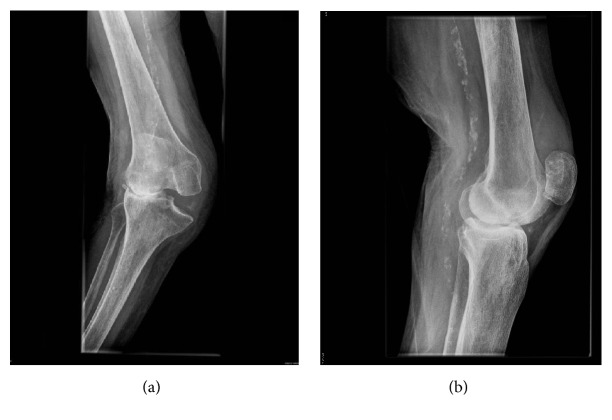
Preoperative standing X-rays of the knee: anteroposterior (a) and lateral (b) view. There is a valgus alignment of 47° (grade III valgus osteoarthritis). Significant wear and destruction of the lateral condyle.
